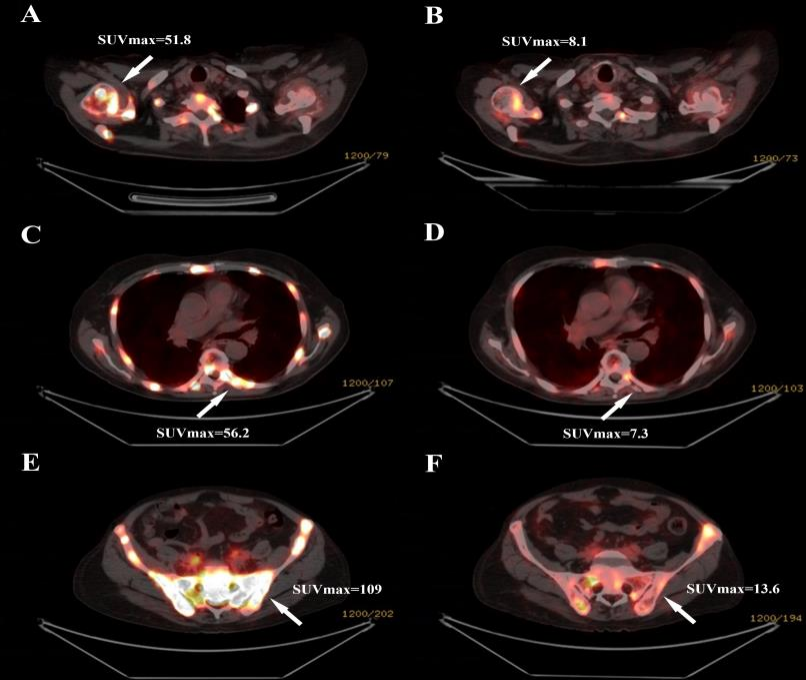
Axial fused images of ^68^Ga-PSMA PET/CT obtained before (A, C and E) the first and after (B, D and F) the sixth cycle of ^177^Lu-PSMA therapy revealed a dramatic response with significantly decreased SUVmax values of metastatic bone lesions (arrows).
